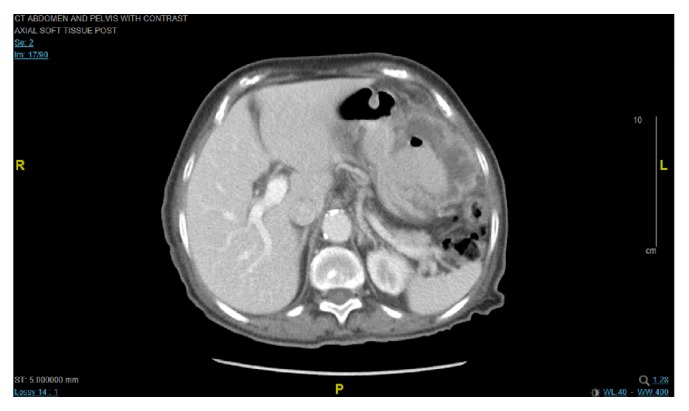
CT abdomen with IV contrast: axial image.
